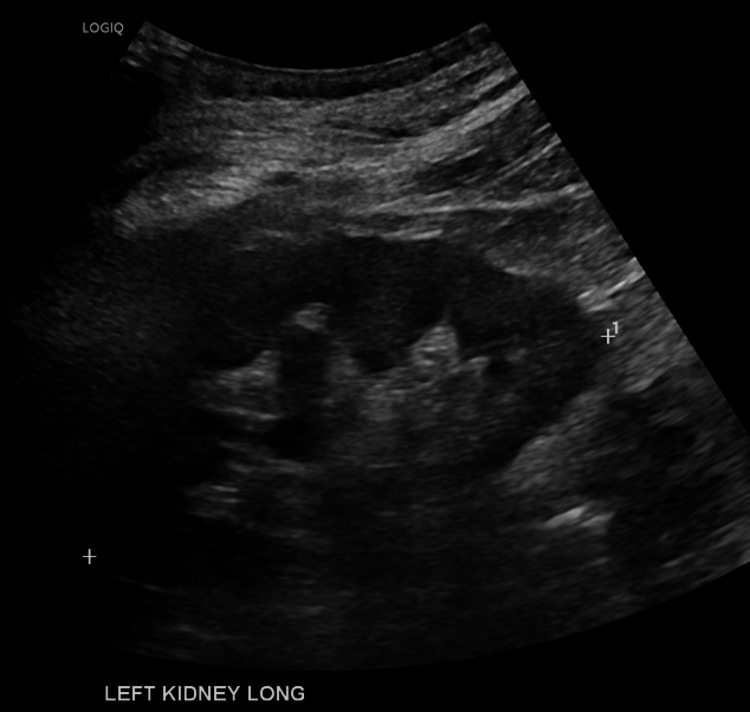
Left Renal Tract Ultrasound The left kidney showing no hydronephrosis
